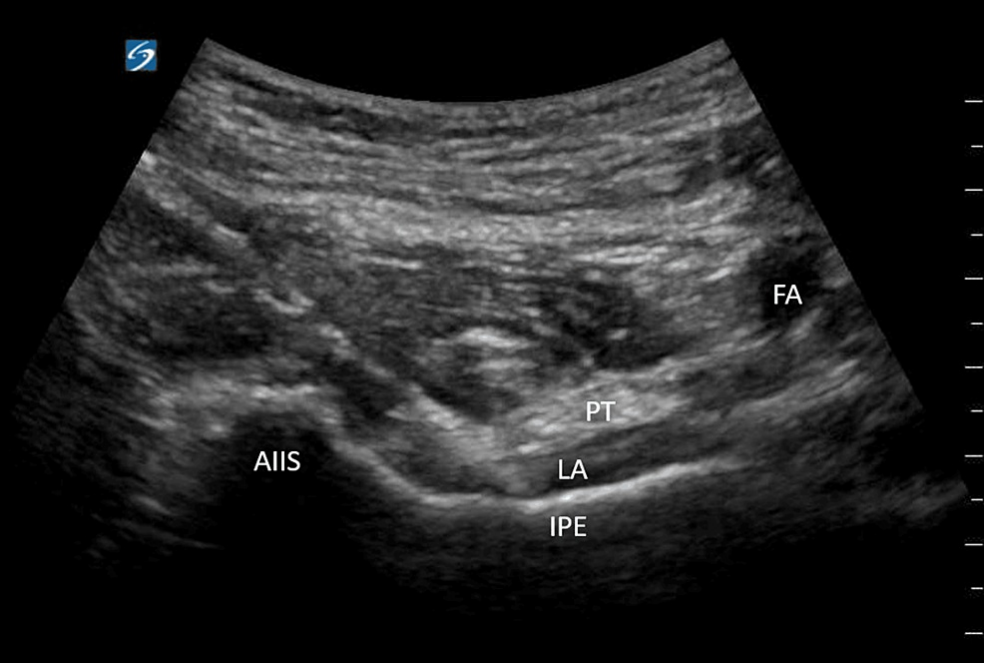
Ultrasound-guided technique for the PENG block AIIS, anterior inferior iliac spine; PT, psoas tendon; FA, femoral artery; IPA, iliopubic eminence; LA, local anesthetic; PENG: pericapsular nerve group
